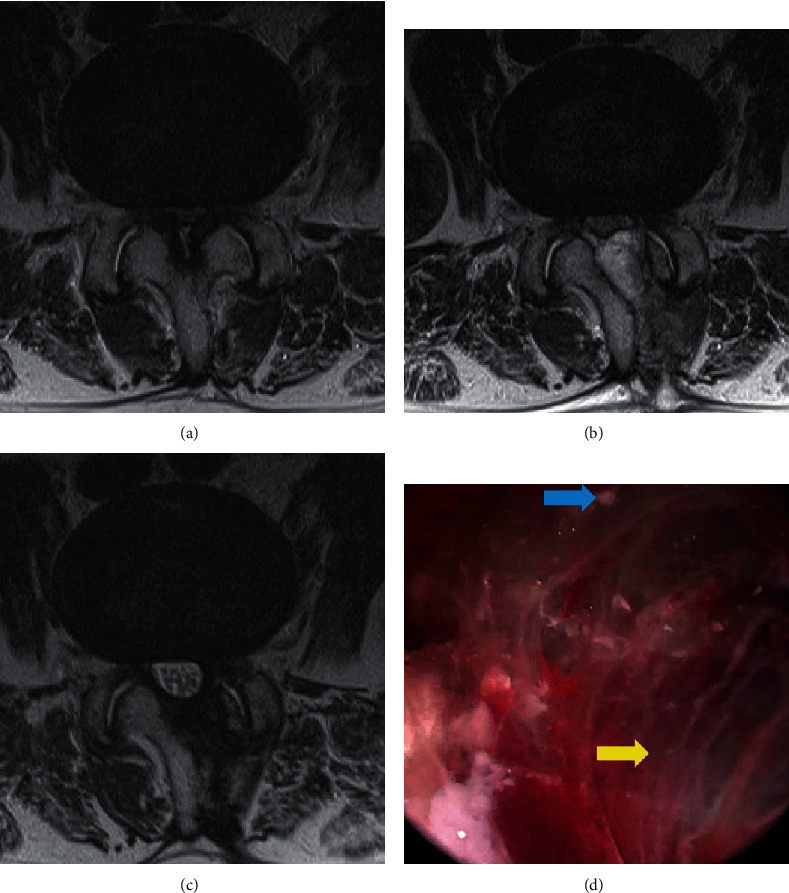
A patient who underwent BESS decompression for L4–5 spinal stenosis and additional revision surgery (hematoma evacuation) due to aggravated neurological symptoms caused by PSEH. (a) Preoperative T2-weighted magnetic resonance imaging showing central stenosis. (b) Postoperative T2-weighted MRI showing grade III PSEH. (c) Follow-up MRI 6 months after revision surgery showing removed PSEHs. (d) Intraoperative image during revision surgery. Yellow arrow: PSEH. Blue arrow: bone debris. (BESS: biportal endoscopic spine surgery, PSEH: postoperative spinal epidural hematoma, MRI: magnetic resonance image).
